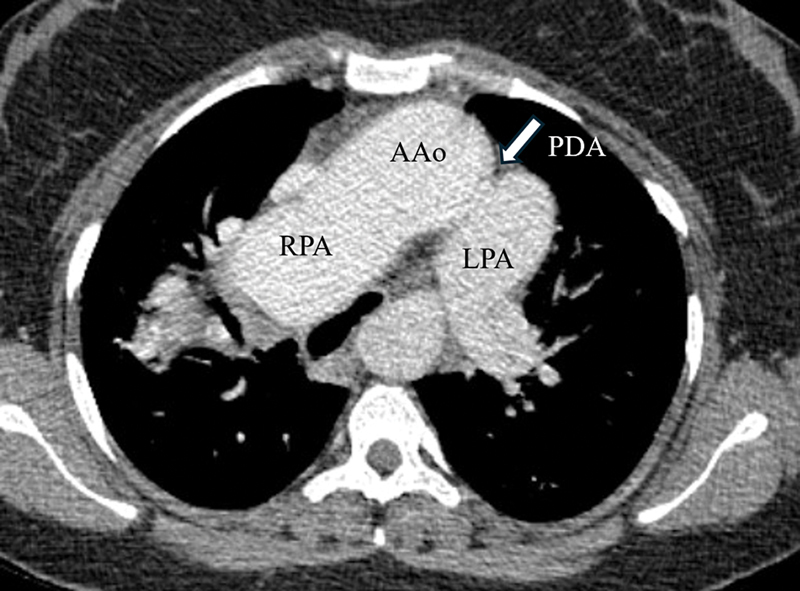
Delayed phase axial computed tomography angiography (CTA) shows anomalous origin of the right pulmonary artery (RPA) from the ascending aorta (AAo). The main pulmonary artery does not bifurcate but continues directly as the left pulmonary artery (LPA). Also noted is the patent ductus arteriosus (PDA, arrow).
